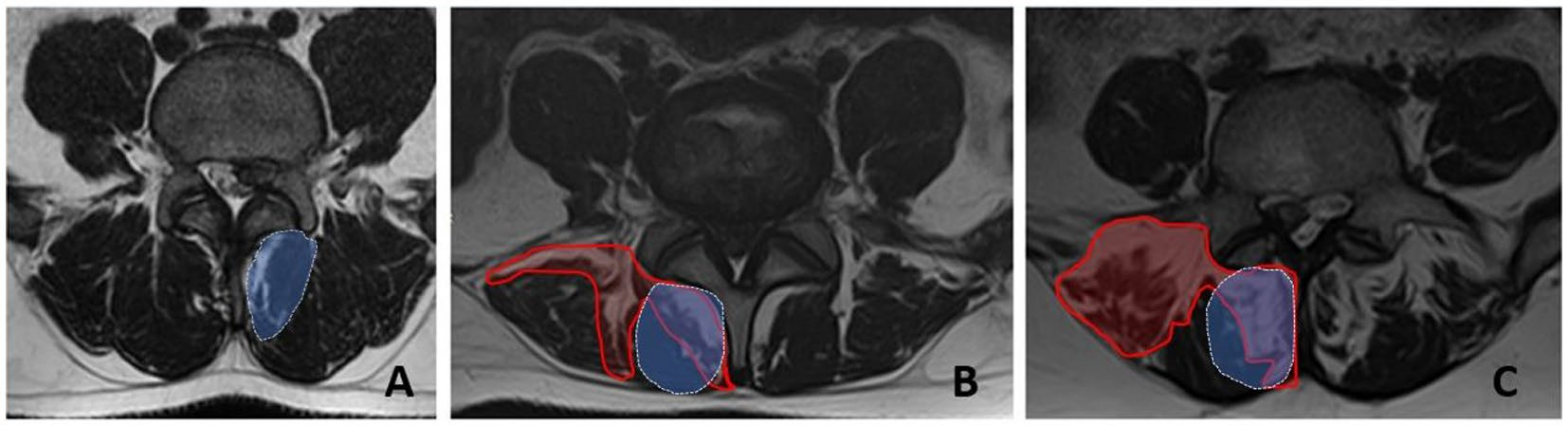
Fatty infiltration grades for the paraspinal muscles at the L5 level measured by MRI (axial sections). A - grade 0, B - grade 1, C - grade 2. The area of fatty infiltration is circled in red. The area of m.multifidus is circled in blue.
